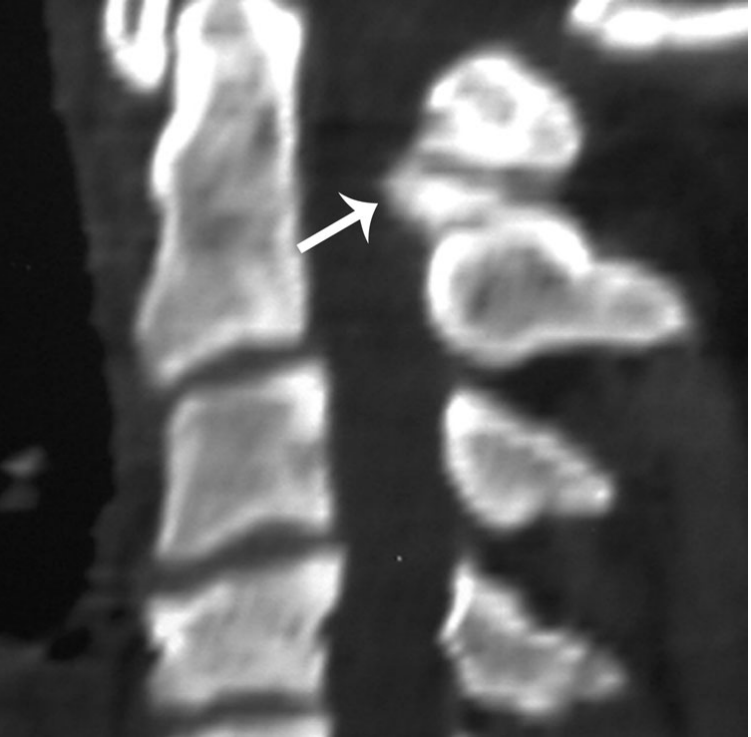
Fig 1 Axial (arrowheads) and Fig 2 sagittal CT demonstrate an expansile lesion (arrow) of the posterior arch of C1. It is contained within the cortex with no soft tissue extension. The bony margins appear smooth, homogeneous and sclerotic.
